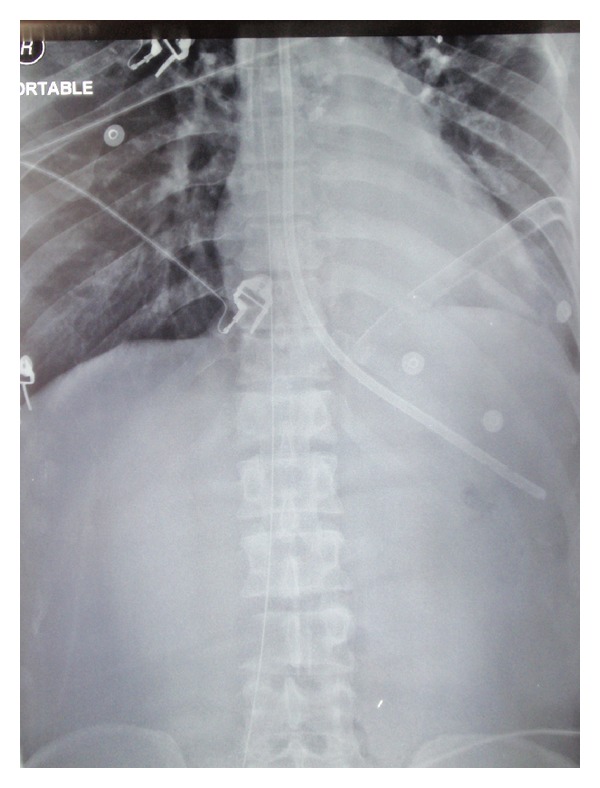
Upper abdominal X-ray showing the lost guidewire extending down the inferior vena cava on the right side of the abdomen.
